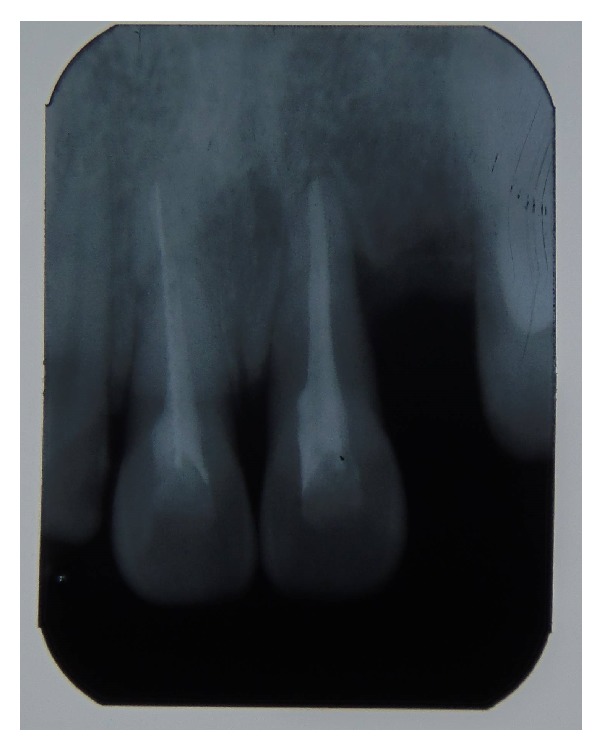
A periapical radiograph after four years follow-up.
